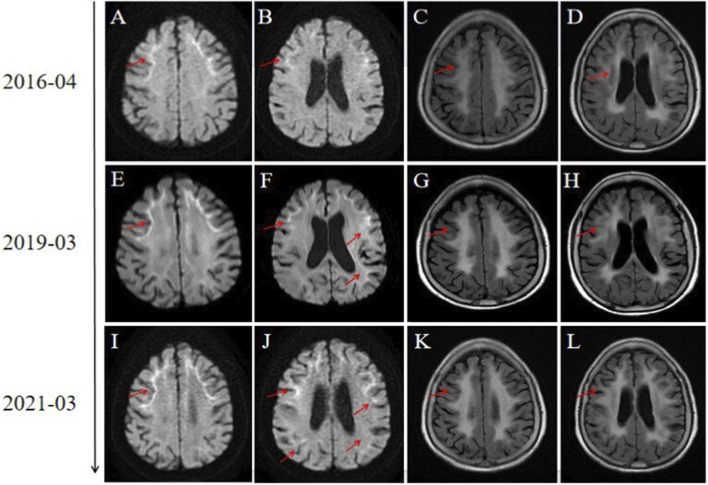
Head MRI findings in neuronal intranuclear inclusion disease (NIID). Panels (A–D), (E–H), and (I–L) correspond to the DWI and the corresponding T2/FLAIR images of the patient in April 2016, March 2019, and March 2021, respectively. Panels (A,B), (E,F), and (I,J) display symmetric, line-like high signals on diffusion-weighted imaging (DWI), indicative of the “insular ribbon sign” (red arrow). Panels (C,D), (G,H), and (K,L) show extensive white matter lesions on T2/FLAIR (red arrow). Comparative findings reveal disease progression from 2016 to 2021.
